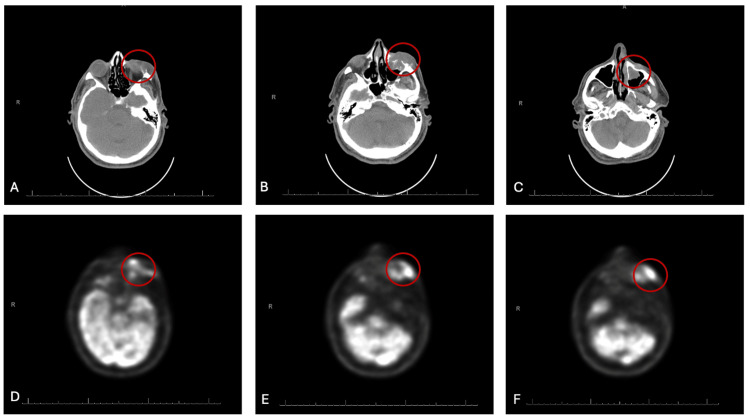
PET-CT of the Head. High-grade, hypermetabolic (D, E, F), infiltrative mass (A, B, C) centered on the left orbit, extending into the maxillary sinus, with destruction of mid and lower orbital bones and both superficial and deep soft tissue involvement.
